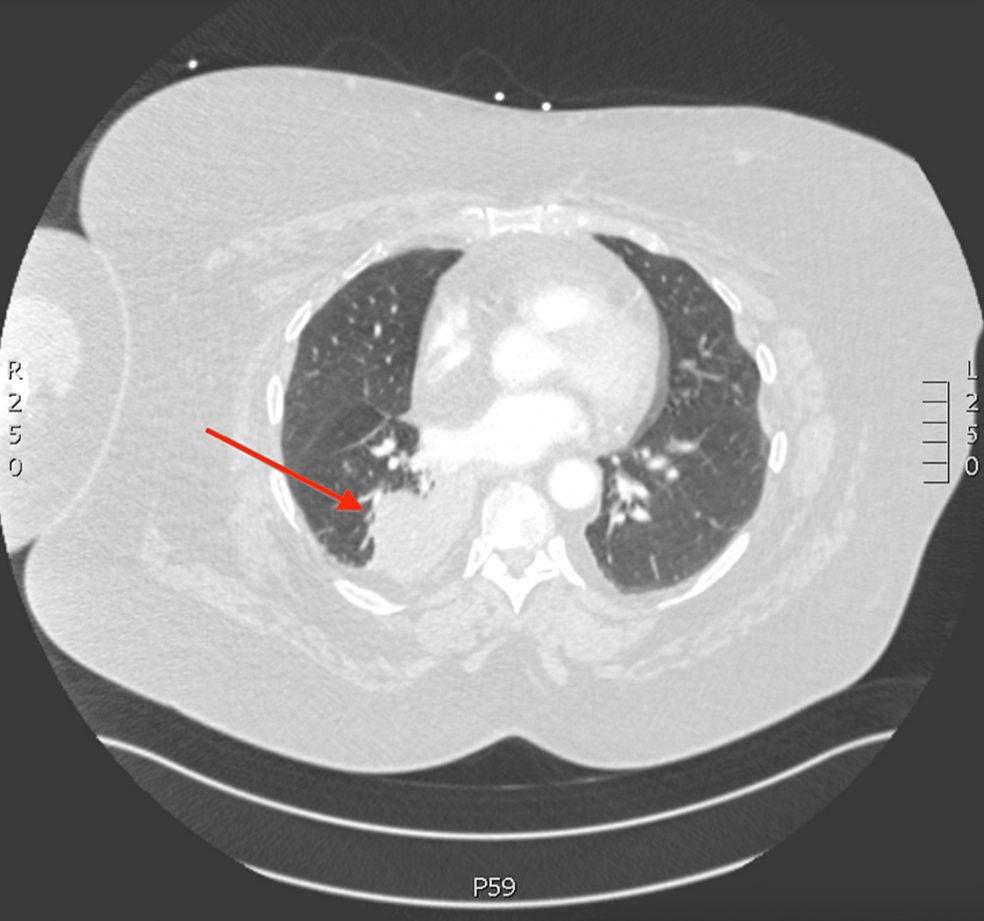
CT Chest with IV contrast revealed atelectatic lung surrounding a right peri-mediastinal mass (red arrow)
